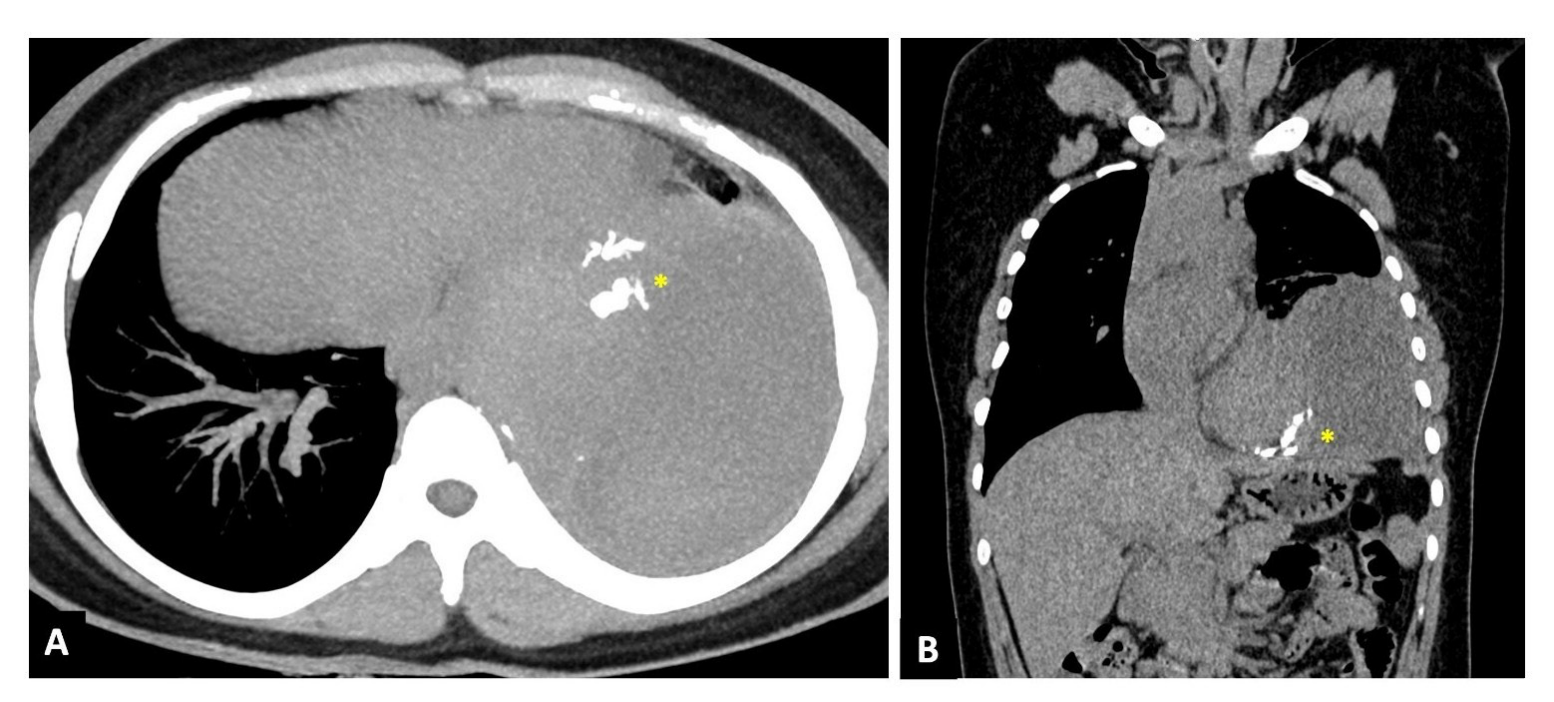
Non-contrast computed tomography (NCCT) axial in maximum intensity projection image (Panel A) and coronal image (Panel B) show glue cast in the pseudoaneurysm sac and inferior phrenic artery (Yellow asterisk) with large surrounding hematoma.
